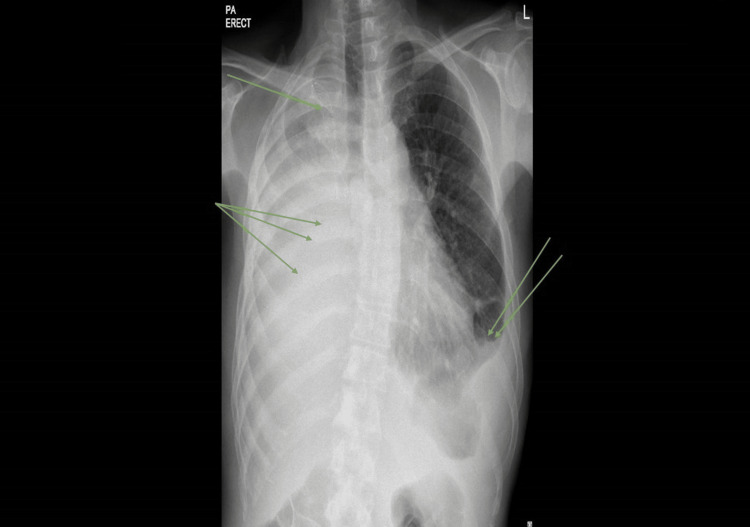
Posteroanterior (PA) erect chest radiograph The PA chest X-ray demonstrates significant opacification of the right hemithorax, indicative of the primary mediastinal mass. The left lung remains clear without evidence of consolidation, though a mild left-sided pleural effusion is present. The single green arrow identifies the metallic stent within the superior vena cava (SVC), previously placed to manage radiation-induced fibrosis. The double green arrows highlight the left-sided pleural effusion, and the triple green arrows delineate the extensive right-sided mass responsible for the hemithorax opacification and extrinsic cardiac encasement.
